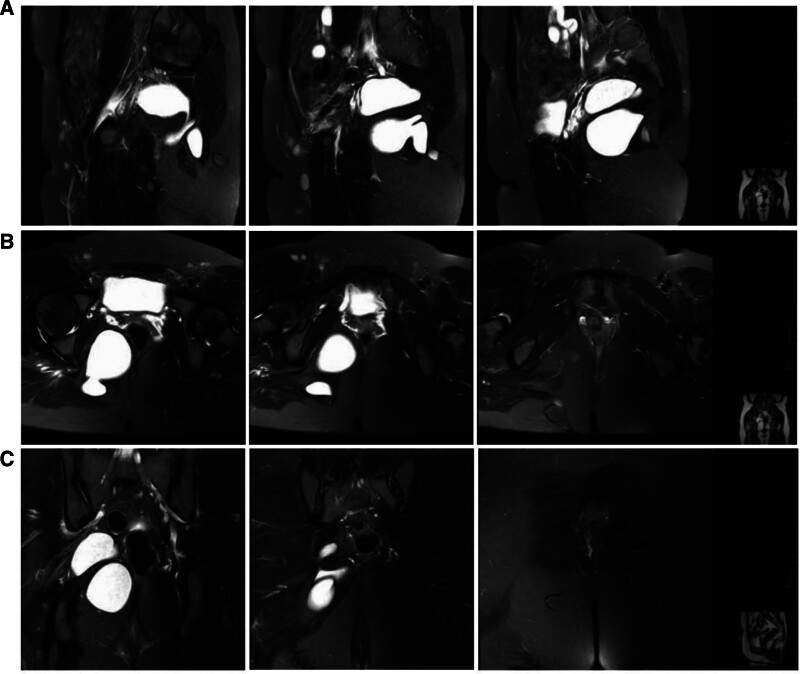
MR images of a retroperitoneal pelvic floor teratoma: sagittal view (A), cross-sectional view (B), and coronal view (C). MR = magnetic resonance.
